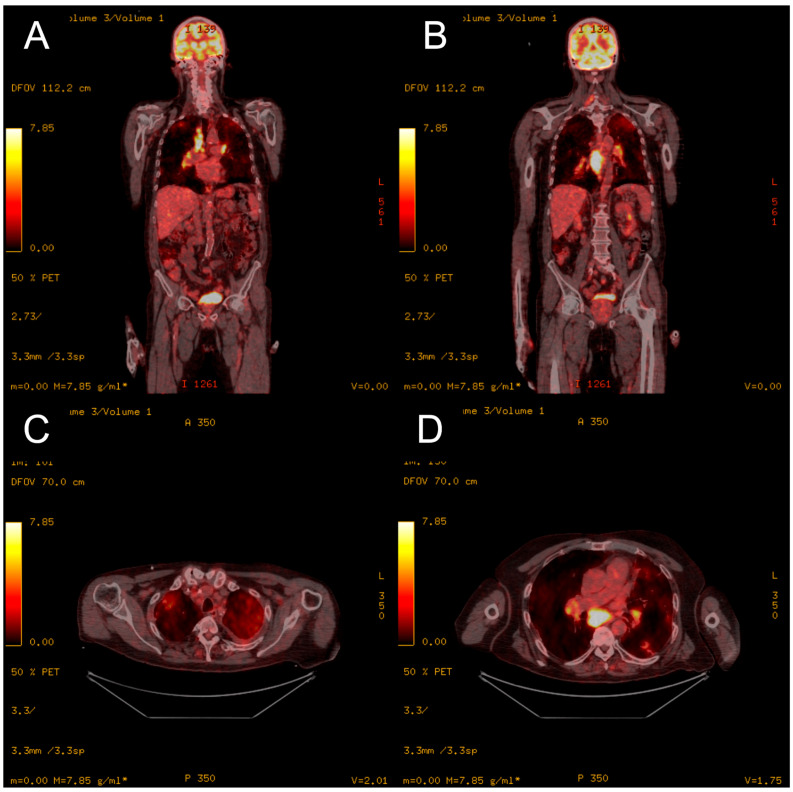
[¹⁸F]Fluorodeoxyglucose PET/CT from 2021 of the coronal plane (A,B) and axial plane (C,D), showing metabolically active mediastinal and hilar lymphadenopathy and pulmonary infiltrates.
